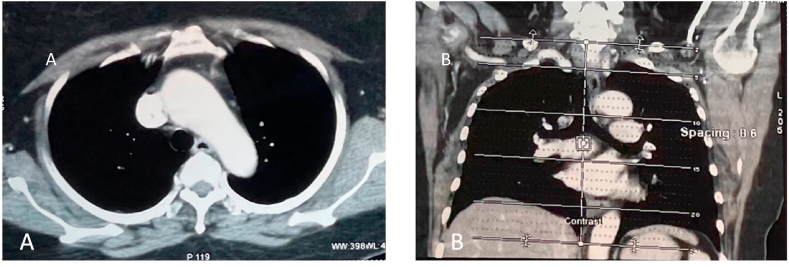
Follow up contrast-enhanced CT in 20^th^ February 2020 (a) axial view (b) coronal view. Figures (a) and (b) showed normal appearance of the mediastinal window with no sign of tumor occurrence or any other abnormalities.
